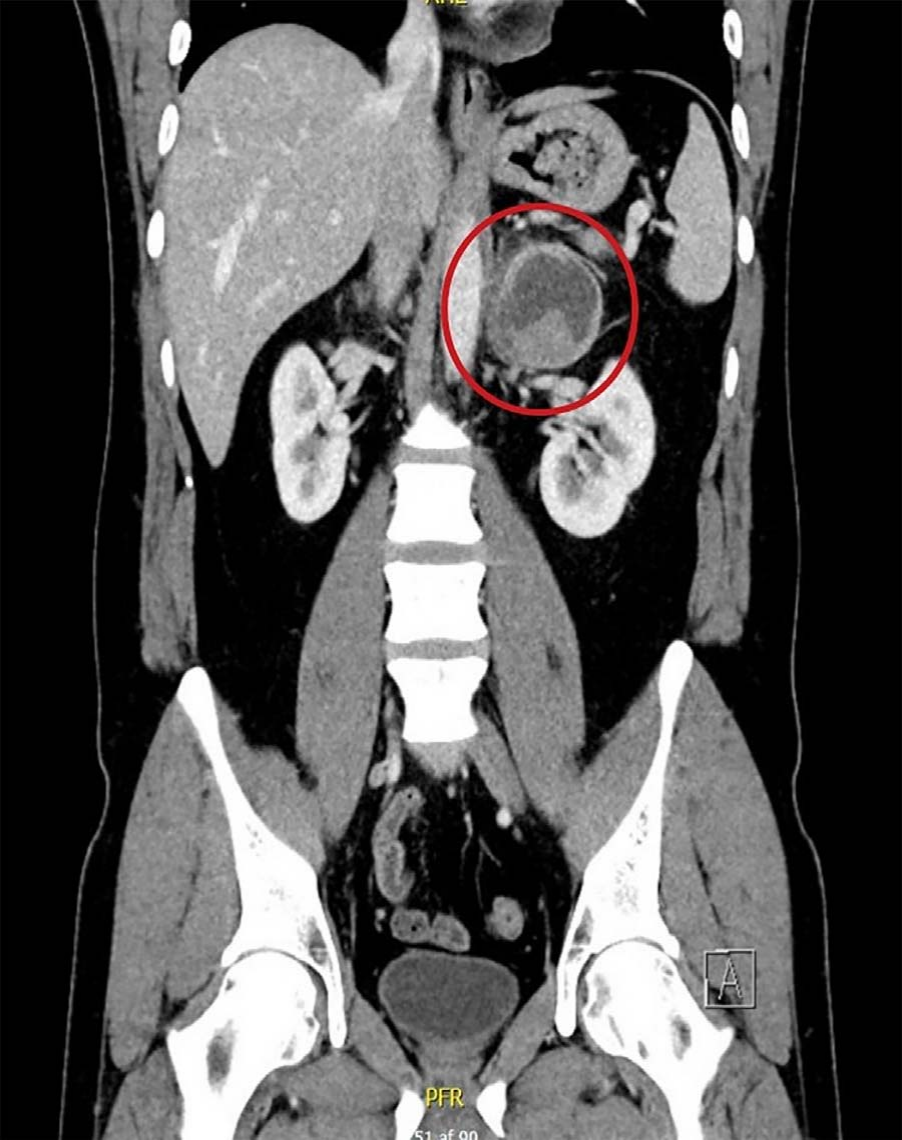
CT scan under admission. Left-sided tumor highlighted inside red circle.
